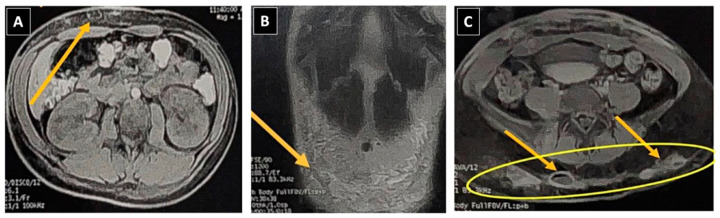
Magnetic Resonance Imaging (MRI) of the pelvic region. (A,B) Large collection in the hypogastrium measuring 12.3 × 2.0 cm, indicated by yellow arrows. (C) Abscesses in the gluteal regions, sizing at 3.5 × 2.0 cm on the right side and 4.2 × 2.4 cm on the left side, accompanied by a slight presence of free fluid within the pelvic cavity.
